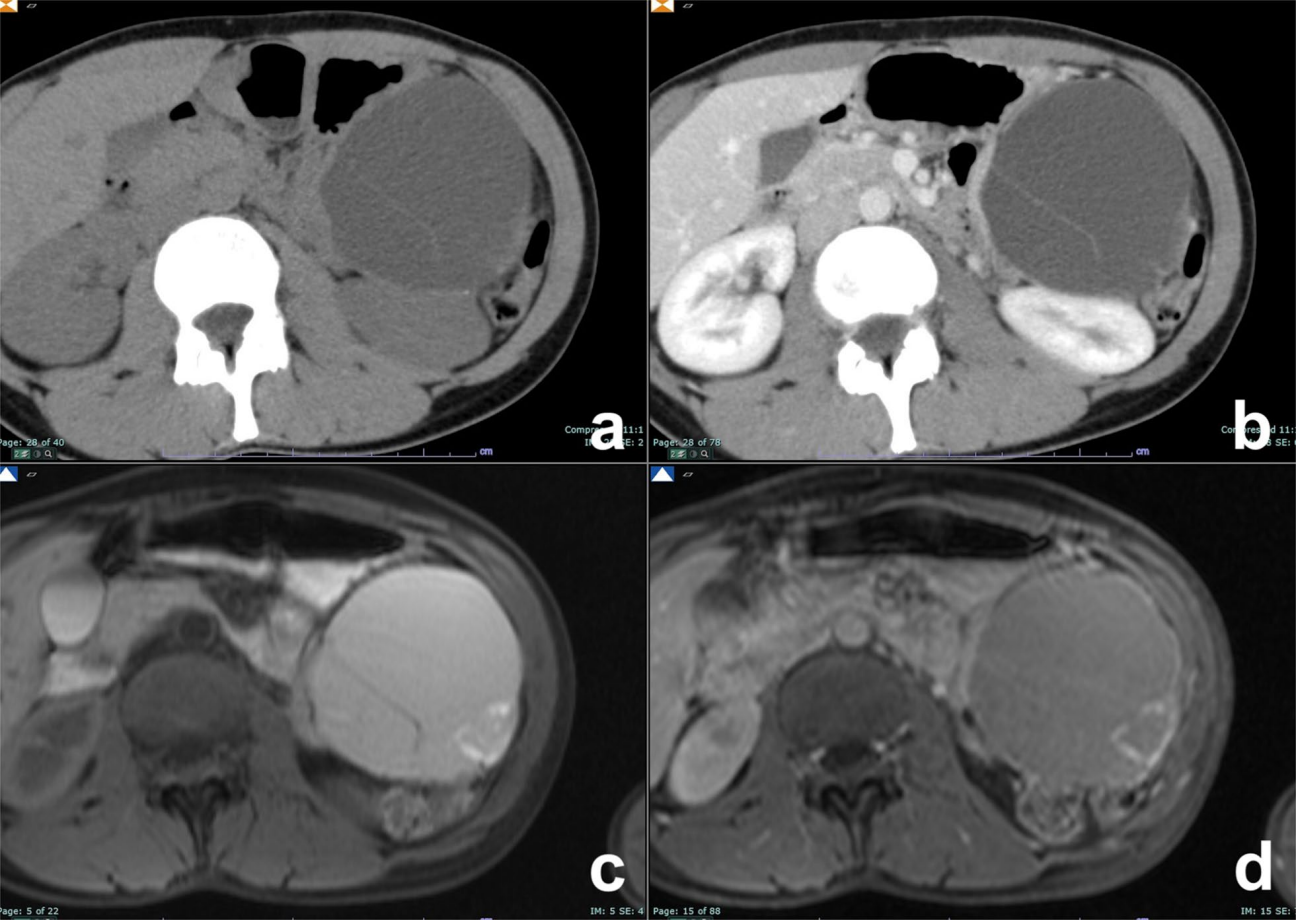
Mucinous cystadenoma, multilocular pattern. At unenhanced CT (a), a large cyst with a tiny septum is visible in the tail of the pancreas, which shows a slight enhancement after contrast media administration (b). On T1w fat-saturated GRE image (c), some hyperintense foci are visible in the inferolateral aspect of the cyst, which do not show significant enhancement after injection of paramagnetic contrast media (d), related to mucin content
